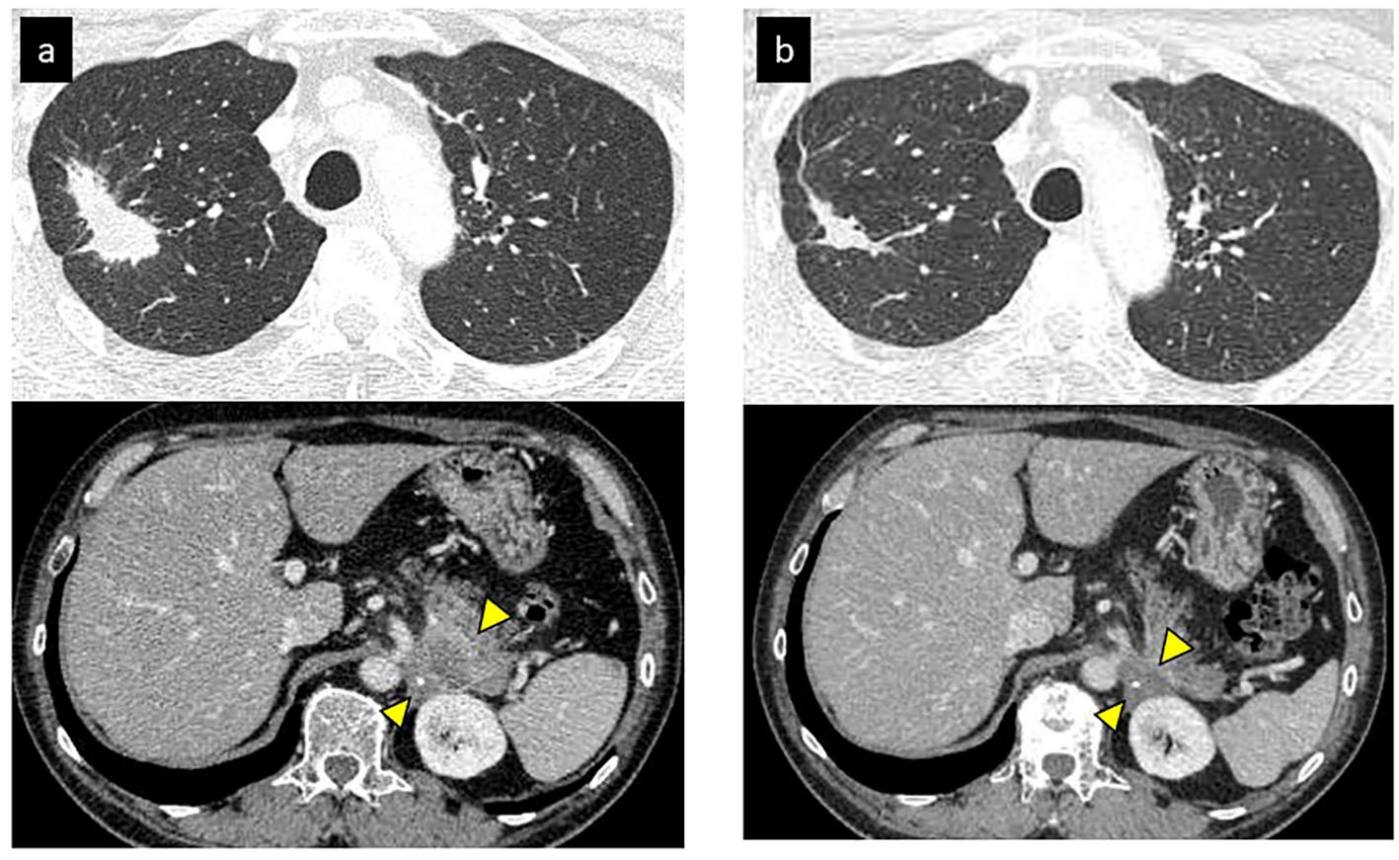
Initial tumor response of nivolumab (partial response). (A) CT revealed a primary lesion in right upper lobe and metastases of left adrenal gland before nivolumab. (B) Primary lesion and adrenal gland metastasis shrunk after 2 months of nivolumab.
